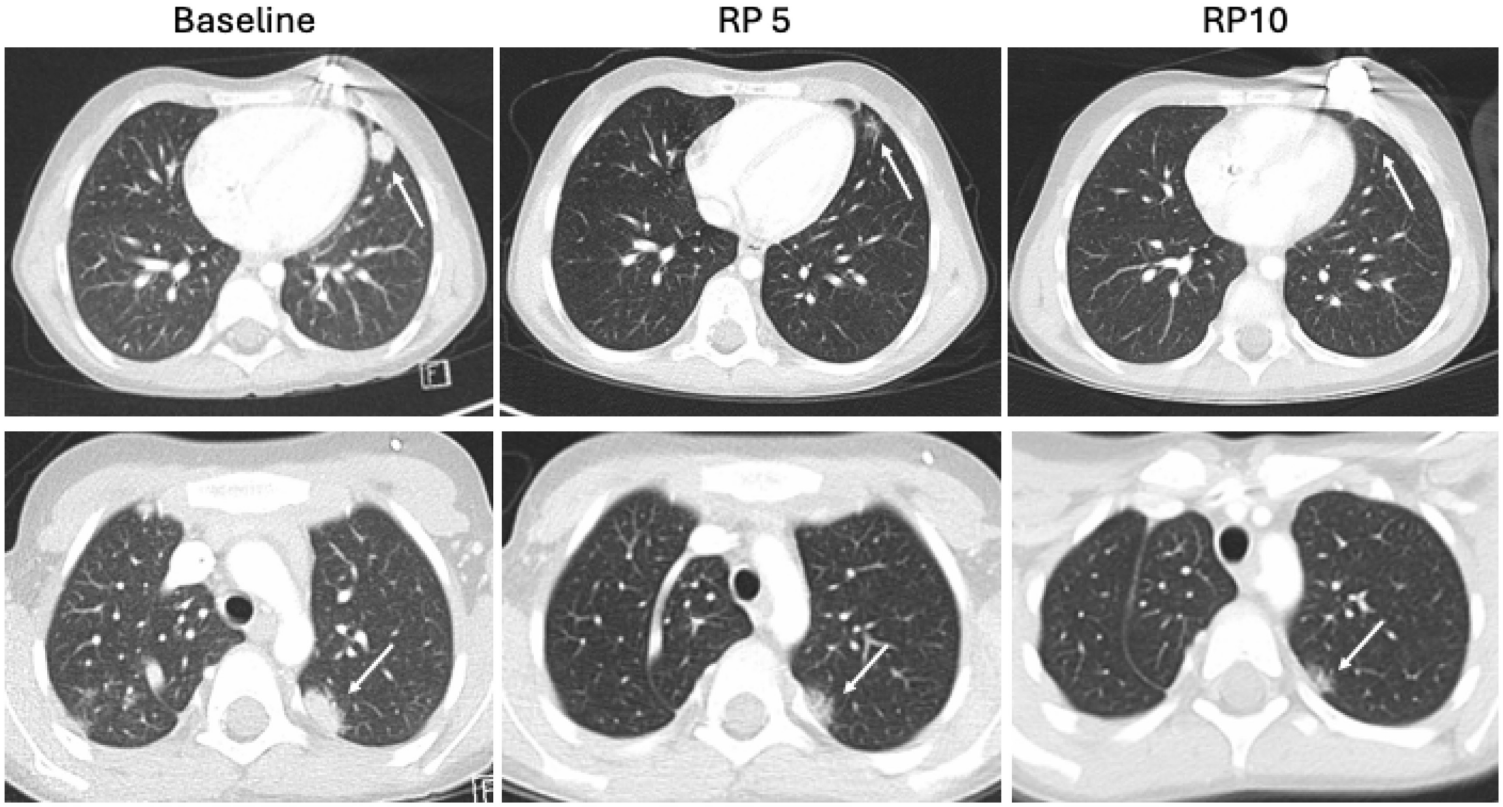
Computed tomography scan from a patient with Ewing Sarcoma treated on DL1 (*Nab*‐sirolimus [35 mg/m^2^/dose] in combination with irinotecan and temozolomide) demonstrating a confirmed partial response. RP = reporting period (cycle number).
